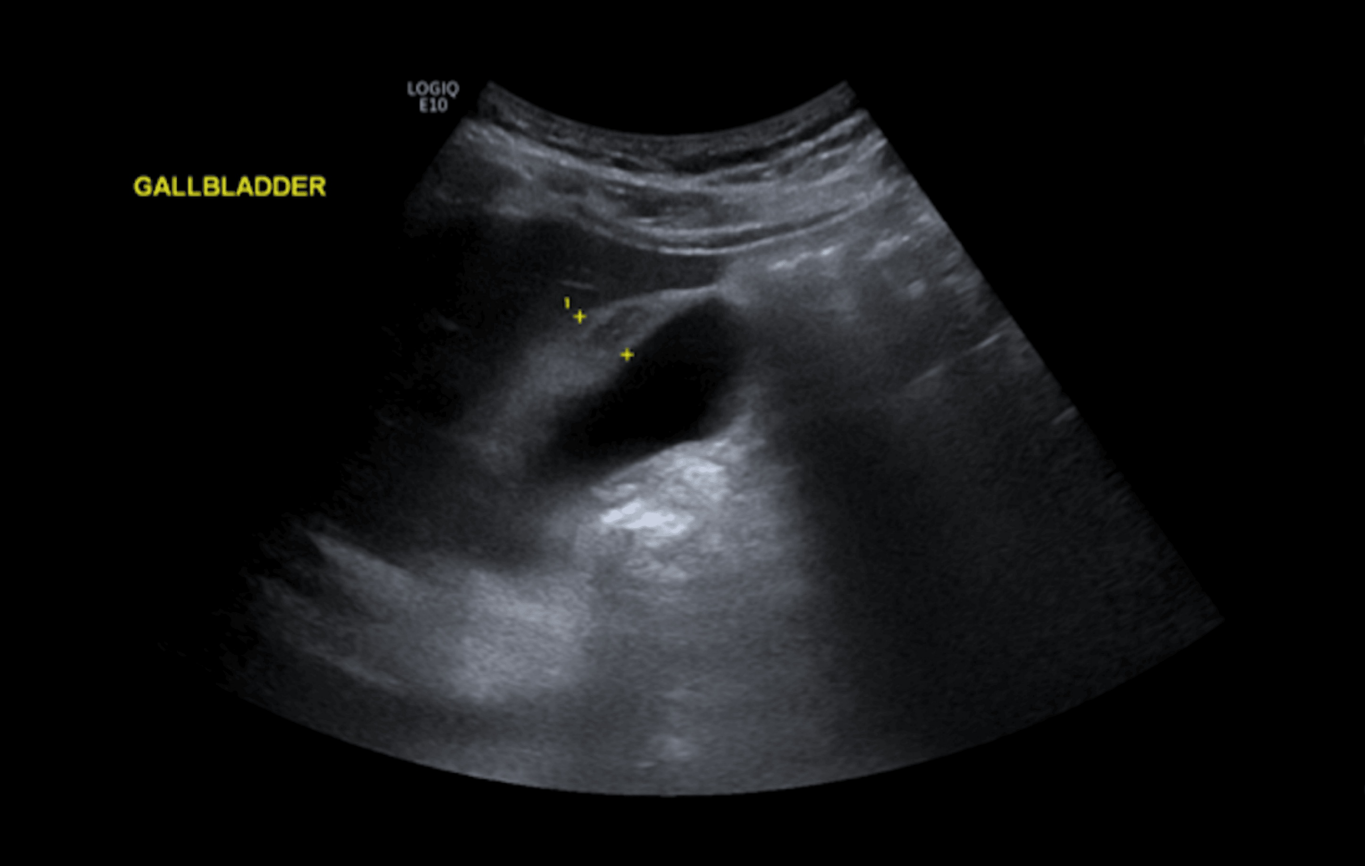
Ultrasound showing a focal thickening of the gallbladder, with no evidence of calculi, consistent with acalculous cholecystitis Gallbladder thickening of approximately 11 mm, as measured between the yellow crosses.
